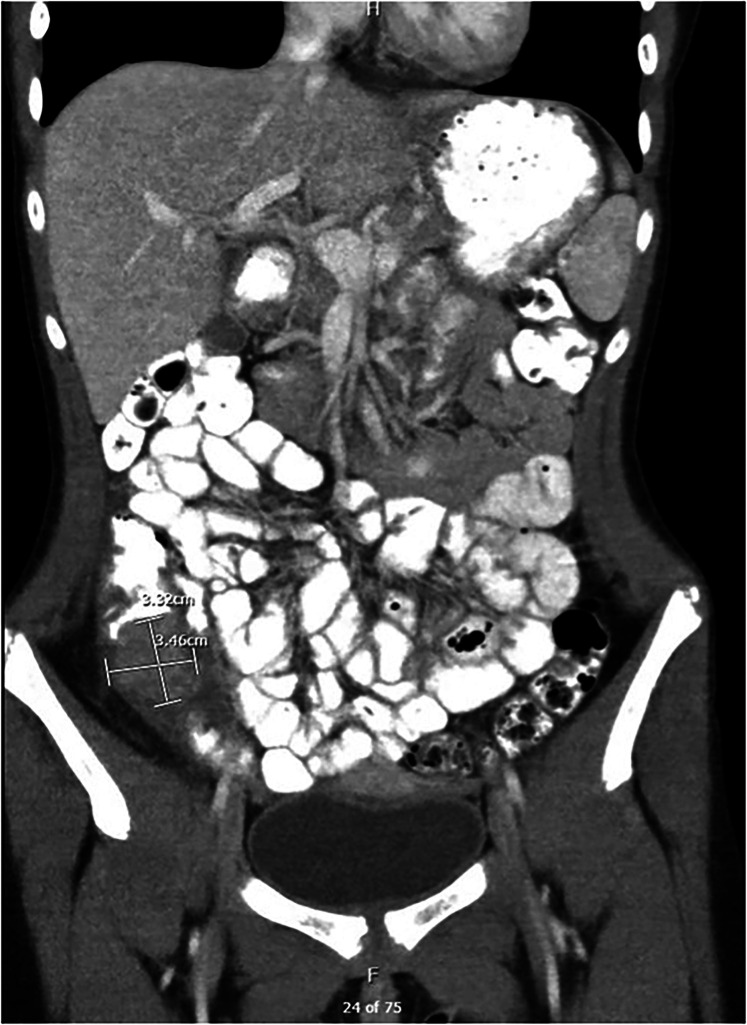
CT scan: intraluminal mass.
